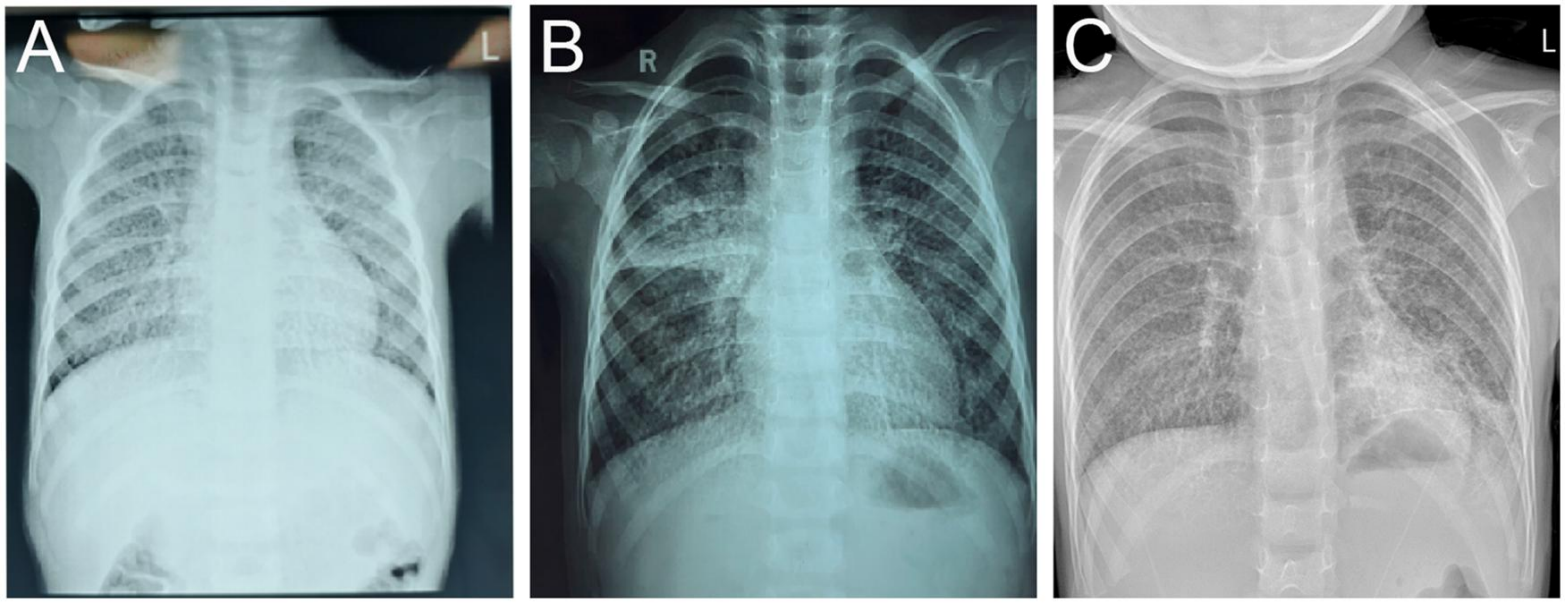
(A) August 2023. The patient presented to an external hospital with fever, cough, and rhinorrhea. Chest radiography revealed increased opacity in both lung fields with small patchy opacities distributed along bronchial patterns, suggestive of bronchopneumonia. The outpatient physician administered azithromycin followed by cefuroxime, leading to symptomatic improvement, but the condition was prone to recurrence. (B) October 2023. The patient presented to another hospital with fever and cough. Chest radiography revealed diffuse interstitial changes with localized consolidation in both lungs, clinically diagnosed as bronchopneumonia. The outpatient physician administered intravenous ceftriaxone sodium and oral azithromycin for infection control, resulting in symptomatic improvement but incomplete resolution. (C) Chest radiography obtained upon current admission.
